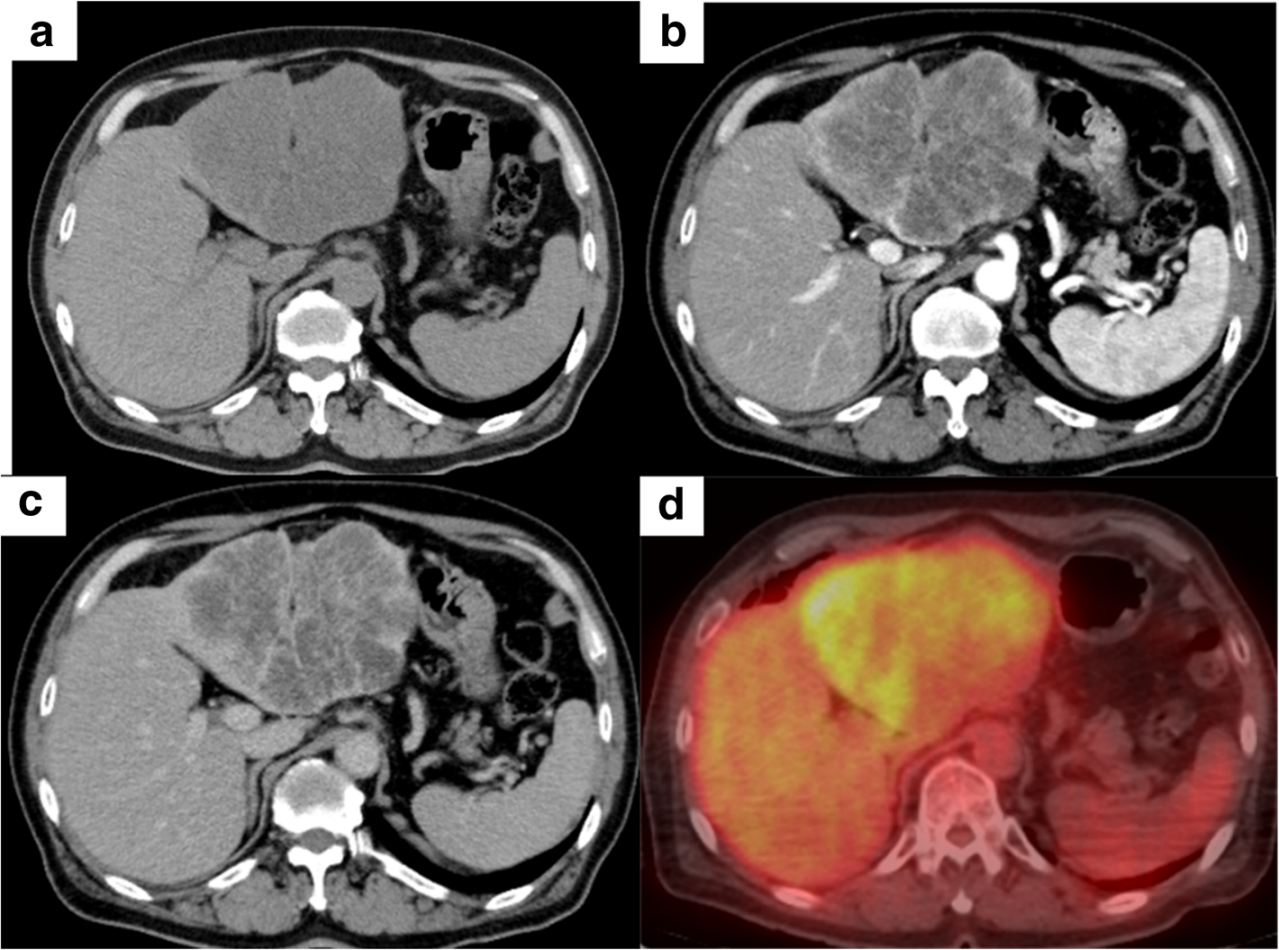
a Plain computed tomography (CT) scan showed a 13.6-cm low-density mass in left hepatic lobe. b Dynamic CT arterial phase scan shows enhanced edge of the mass with non-enhanced interior. c Low-density area remained in the equilibrium phase. d Fluorodeoxyglucose-positron emission tomography (FDG-PET) demonstrated a high FDG uptake (SUVmax 4.9) in the peripheral region of the mass
